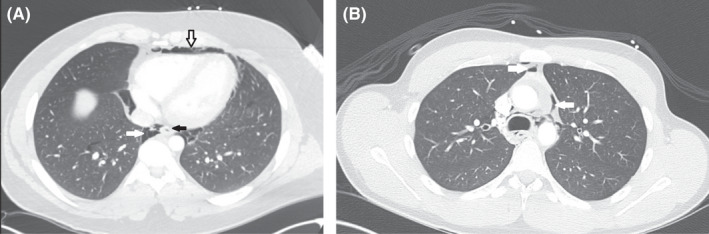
CT Thorax (Axial views) with (A) moderate pneumomediastinum with pneumopericardium (white arrows) and a collapsed esophagus (black arrow). (B) Moderate pneumomediastinum (white arrows), esophagus not well visualized
